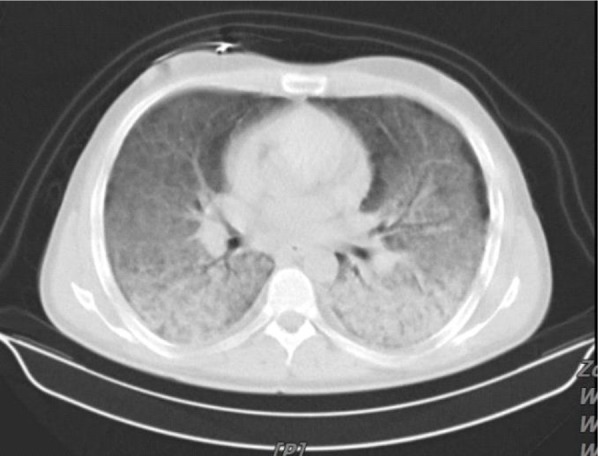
CT- Scan of the chest showed bilateral alveolar infiltration with Air Bronchogram and ground-glass opacification
